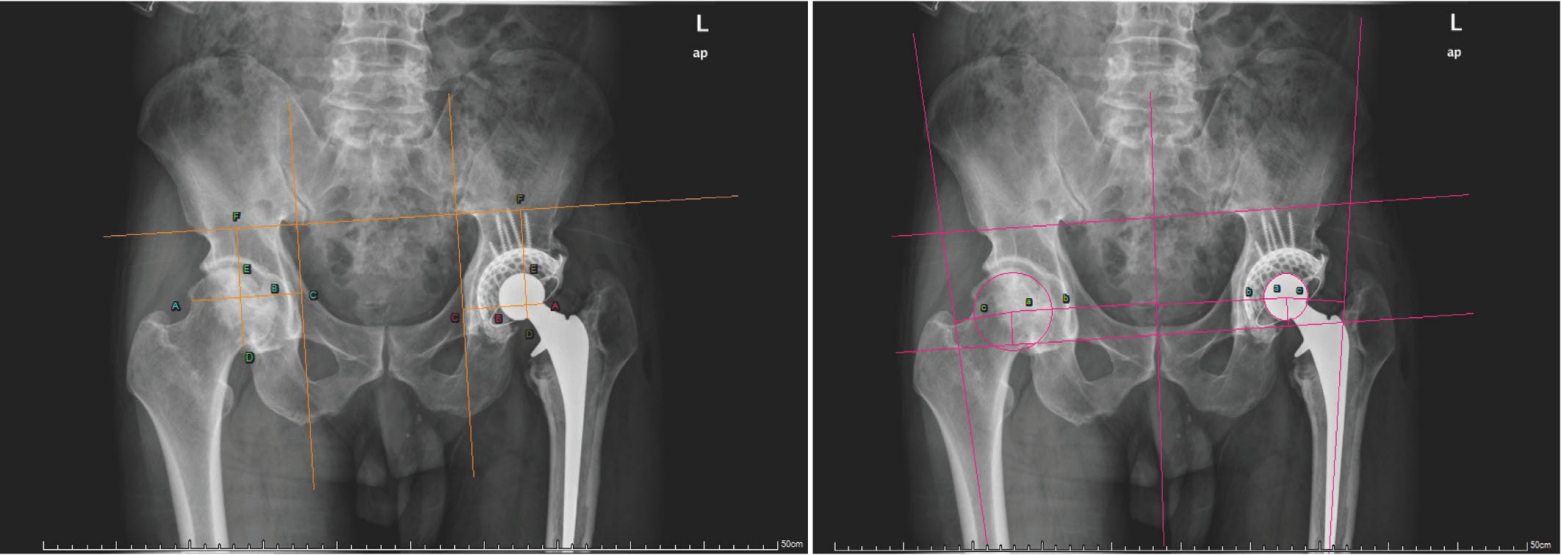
(A) Post‐revision radiographs showing the measurement of horizontal value (AC, BC), vertical value (DF, EF) of the acetabular implant, inclination angle of acetabulum (G); (B) Measurement of offsets in a hip (a: medial offset; b: ilioischial offset; c: femoral offset; d: center of rotation).
